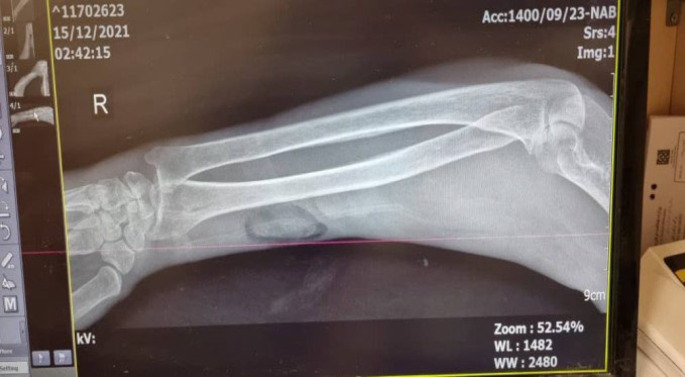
X-rays of the arm showing unremarkable bony abnormality and only showed subcutaneous skin thickening
